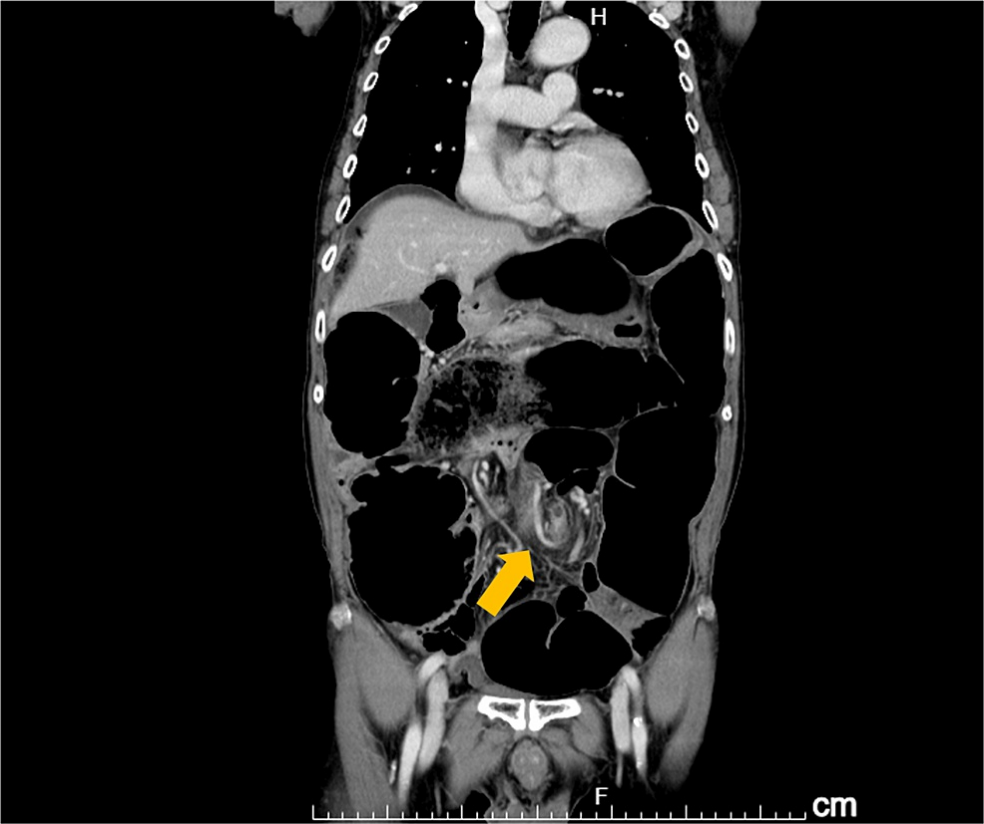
CT imaging indicating sigmoid colon axis torsion at the arrowed location
